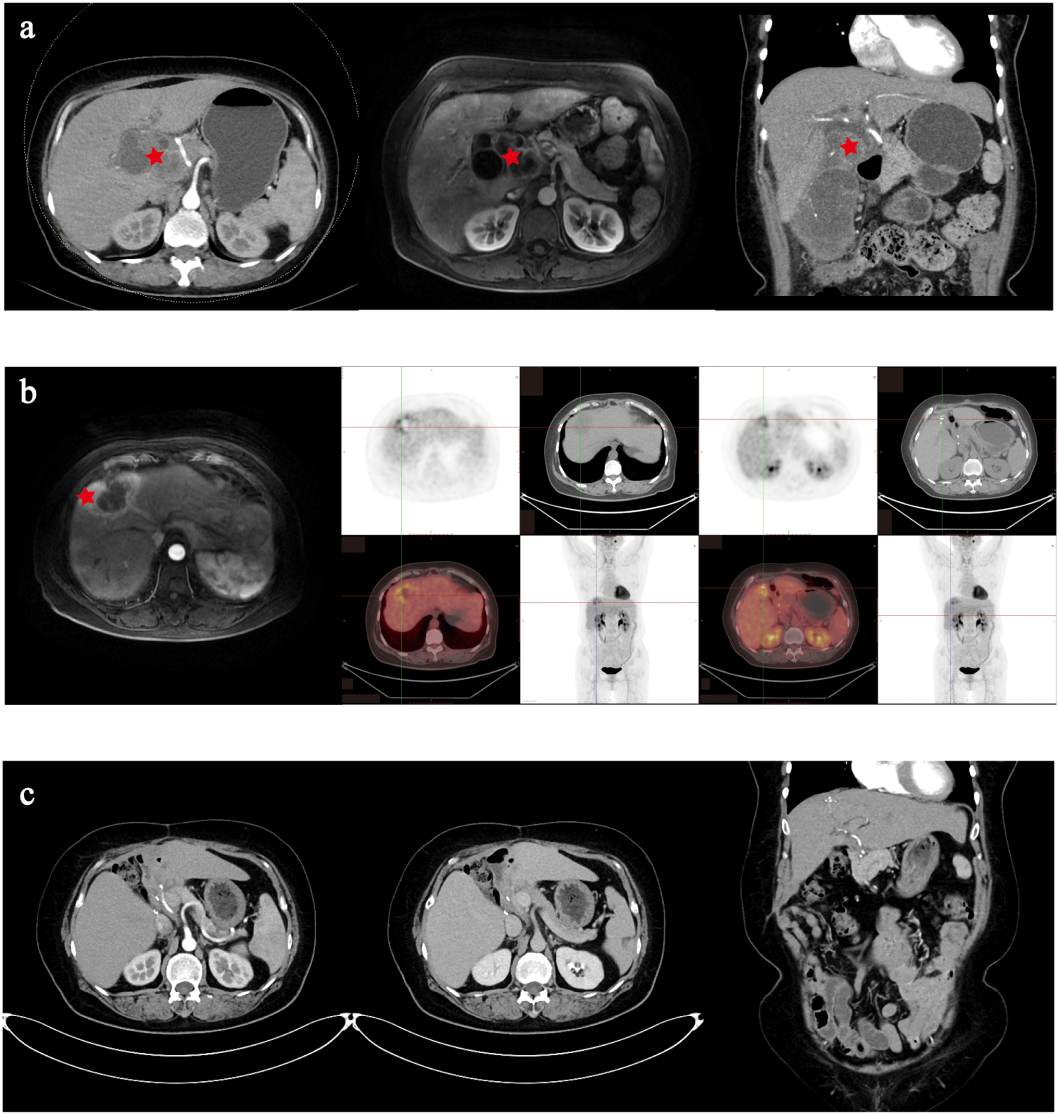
Imaging examination of the patient. (a) Contrast-enhanced computed tomography (CT) and contrast-enhanced magnetic resonance indicating locally advanced gallbladder carcinoma. (b) Positron emission tomography–CT indicating tumor recurrence in the surgical area. (c) Contrast-enhanced CT indicating tumor-free condition at the recent follow-up.
